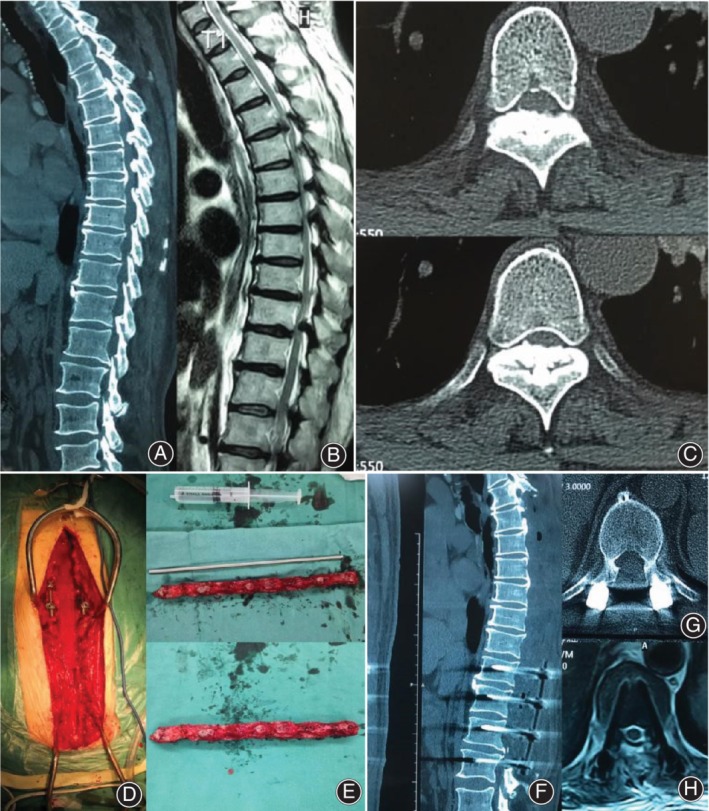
A 58‐year‐old male with numbness and weakness of both lower limbs for 3 years was diagnosed with thoracic spinal stenosis. (A and B) Preoperative MRI T2‐weighted images showed multisegment ligamentum flavum hypertrophy and corresponding spinal stenosis. Preoperative CT sagittal position showed multisegment ligamentum flavum calcification. (C) Preoperative thoracic CT axial position showed ligamentum flavum calcification and significantly reduced spinal canal volume. (D and E) Intraoperative thoracic spinal segment laminectomy resection. (F) Postoperative CT sagittal position chest four lumbar two laminectomy, parallel internal fixation to stabilize the thoracolumbar spine. (G and H) Postoperative thoracic CT MRI showed that the spinal cord was relieved, and the cerebrospinal fluid signal seen before and after the spinal cord was removed.
